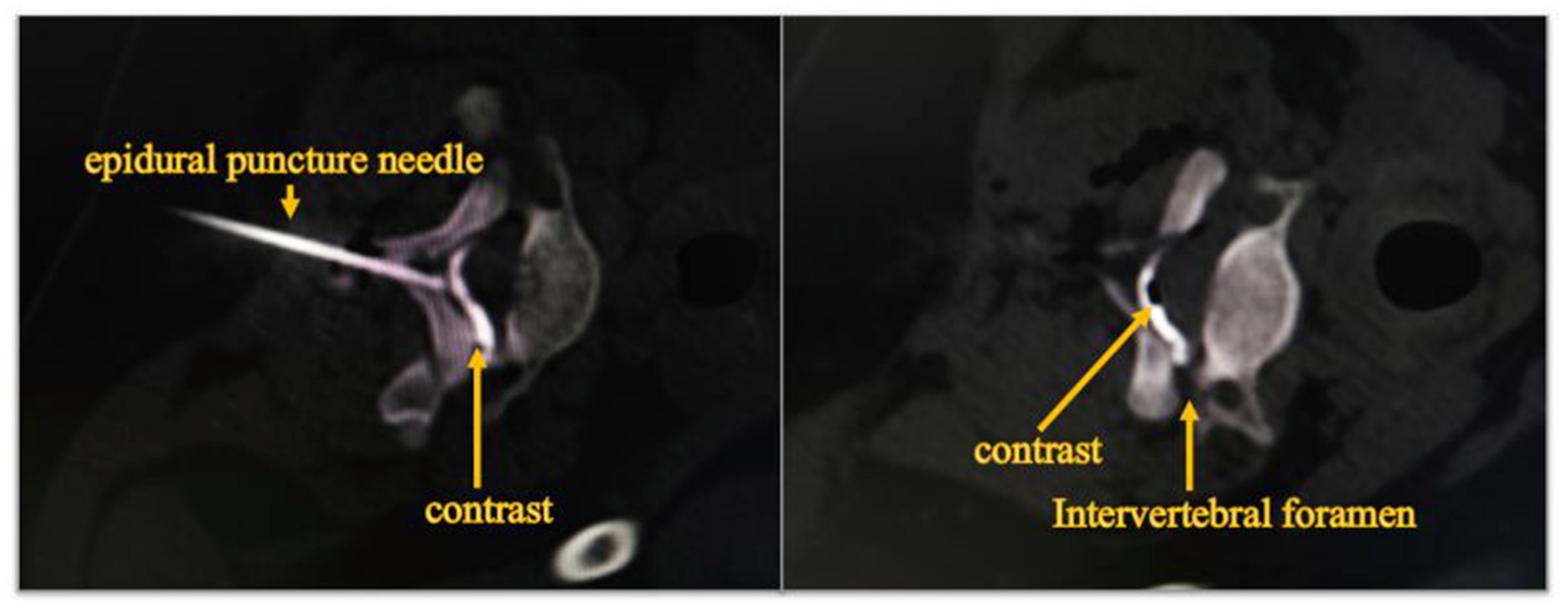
Diffusion of contrast agent in epidural space at CT fluoroscopy. The image shows that the epidural puncture needle reaches the epidural space, and contrast injected into the epidural space spreads outward along the nerve root canal.
